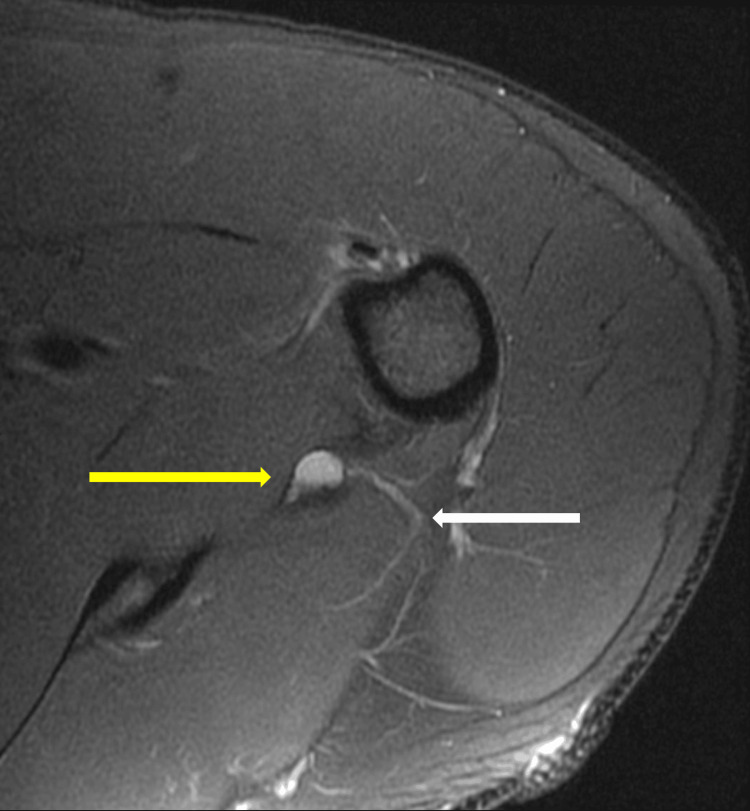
Axial T2 MRI demonstrating the paralabral cyst abutting the axillary nerve within the quadrilateral space. The yellow arrow demonstrates the cyst. The white arrow demonstrates the axillary nerve
